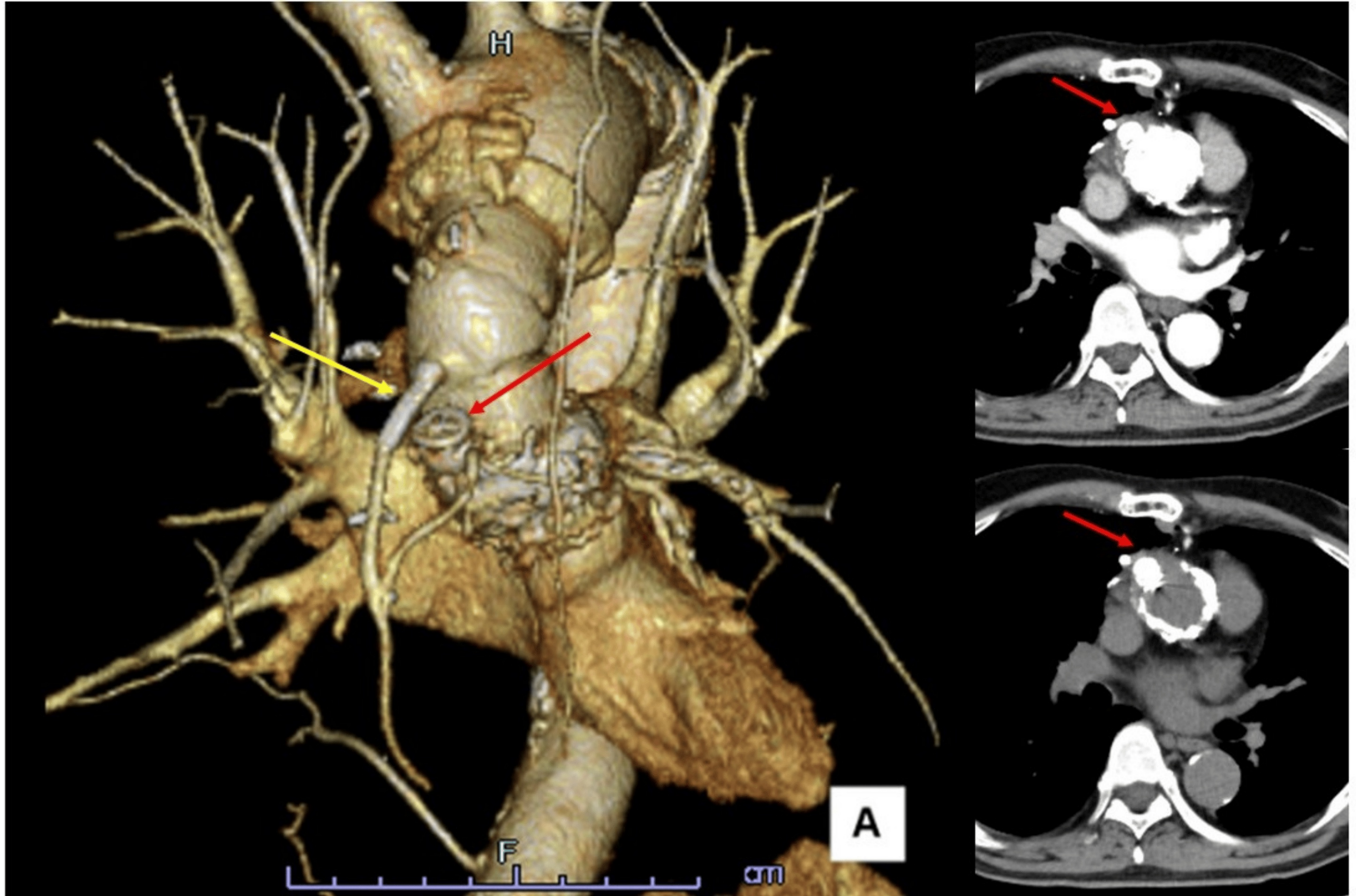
Postoperative CT (3D and contrast-enhanced CT) images demonstrating optimal placement of the stent (yellow arrow) and AVP Ⅱ (red arrow). The high-density structure observed in the contrast-enhanced CT images corresponds to the AVP II. CT: computed tomography, 3D: three-dimensional, AVP Ⅱ: Amplatzer Vascular Plug II
